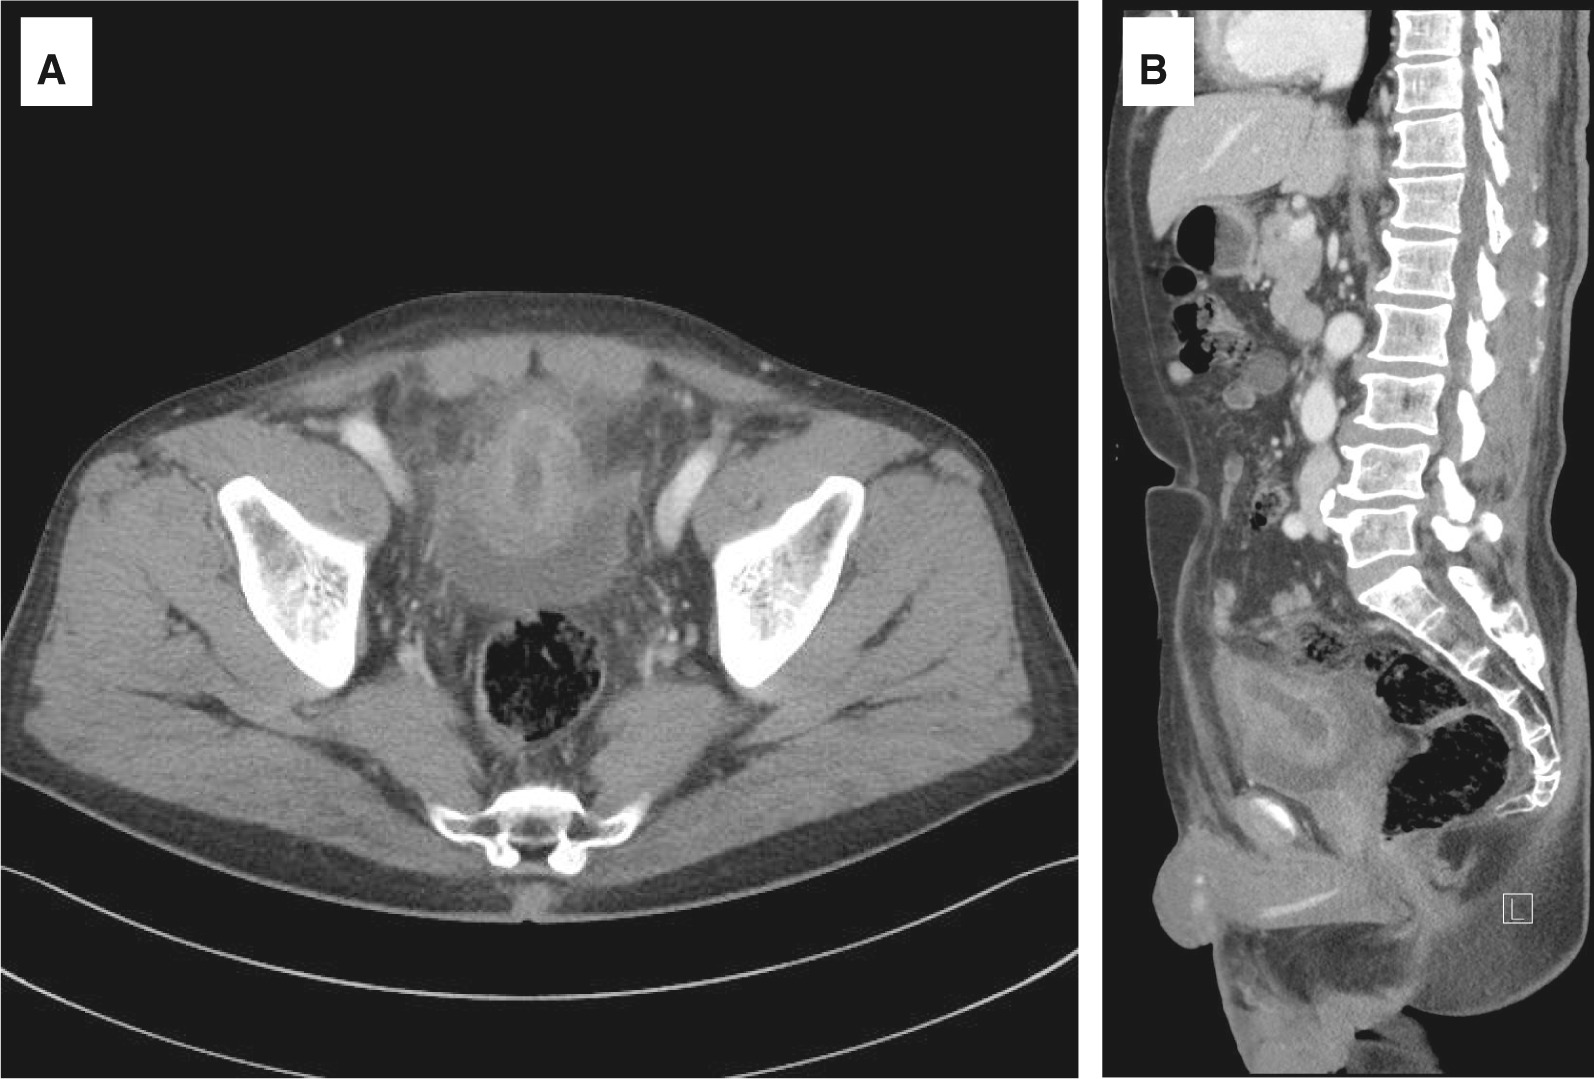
Computed tomography showing a heterogeneous enhancing mass on the anterosuperior portion of the bladder. A Axial view, B sagittal view
